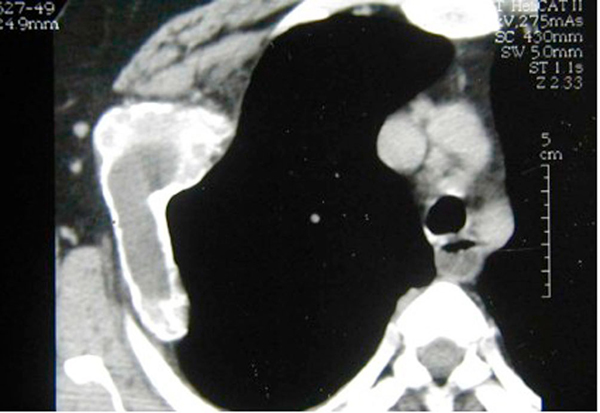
Computed tomography of the lesion showed a multiloculated, expanding mass with fluid levels in the right 3rd rib.
